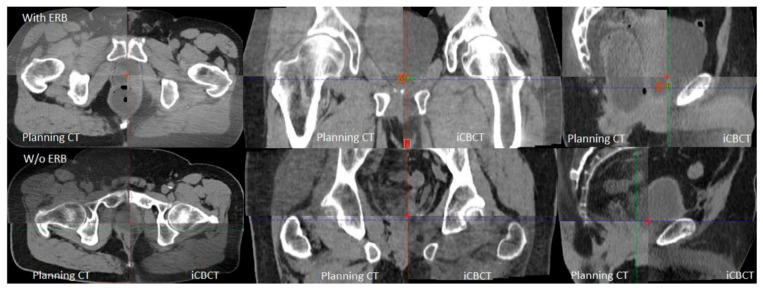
Axial, coronal, and sagittal views demonstrating planning CT and iCBCT image guidance to identify rectal wall and bladder interface and space between bladder and obturator musculature.
